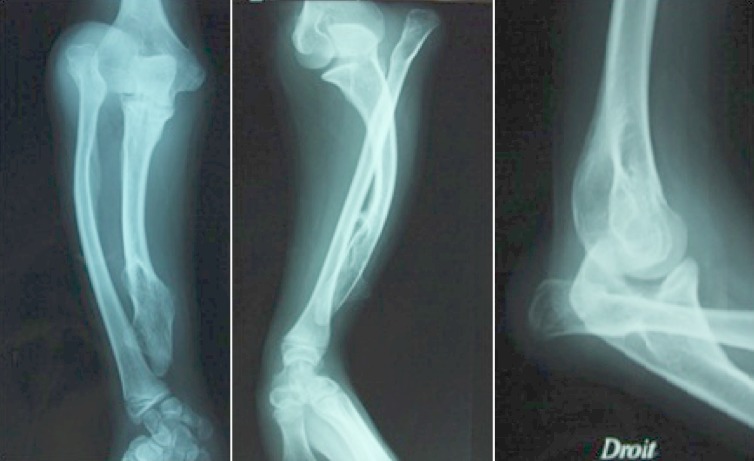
Les radiographies de face et de profil montre l'exostose ulnaire inférieure et la luxation de la tête radiale
